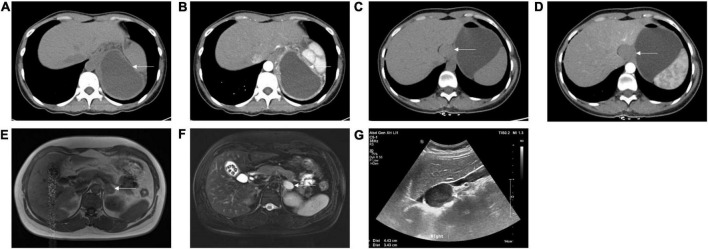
Appearance of subdiaphragmatic bronchogenic cysts on computed tomography (CT), magnetic resonance imaging (MRI), and B ultrasound. (A) Non-enhanced CT showed a low-density cystic lesion between the pancreatic tail, stomach, spleen, and diaphragm from case 13. (B) Enhanced CT showed slight enhancement of the cystic wall from case 13. (C) Non-enhanced CT showed a soft-tissue density lesion adjacent to the lesser curvature of the stomach from case 19. (D) Enhanced CT showed no enhancement of the lesion from case 19. (E) T1 weighted image on MRI showed a hypointense lesion at the left adrenal region from case 16. (F) T2 weighted image on MRI showed a hyperintense lesion at the left adrenal region from case 16. (G) B ultrasound showed a well-defined, hypoechoic lesion at the right adrenal region from case 17. Arrows indicate the cysts.
